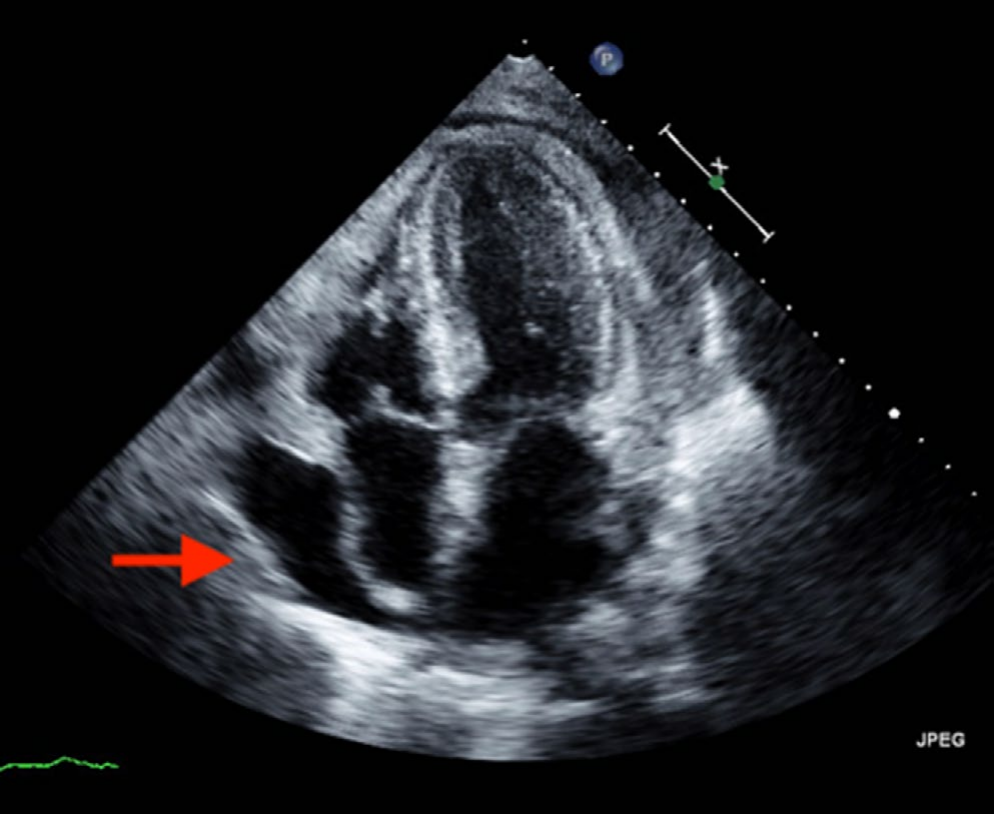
2D echocardiogram apical four‐chamber view. Late diastolic/ early systolic collapse of right atrium suggestive of tamponade physiology (red arrow)
